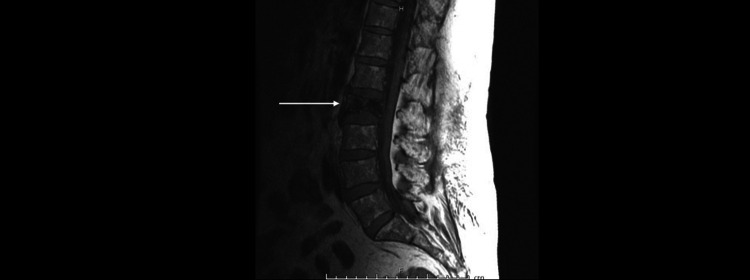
MRI lumbar spine with contrast. The white arrow shows L2 increased heterogeneity and an overall decrease in signal intensity.
